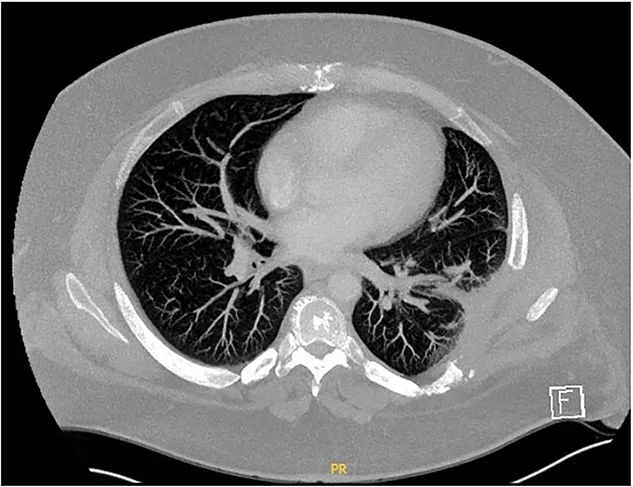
CT scan from May 2023, 5 months after treatment, showing a defect in the chest wall with soft tissue filling and excellent healing of the lung parenchyma.
